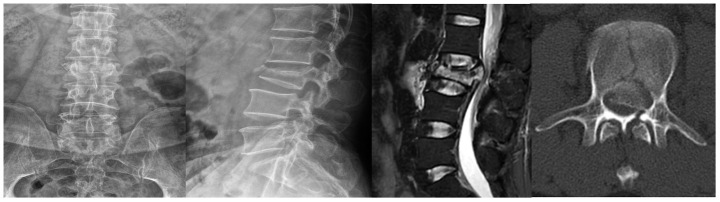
Lumbar X-rays, MRI, and CT examinations of the patient.
